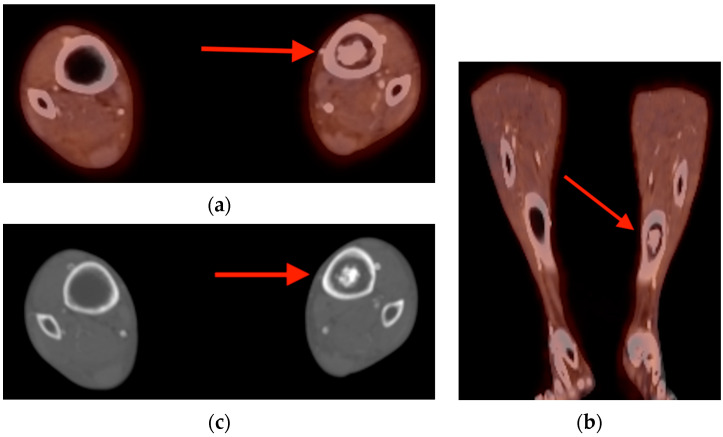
Axial (a) and coronal (b) fused FDG PET/CT images obtained in a 62-year-old man with melanoma showed a coarsely calcified intramedullary bone lesion with mild uptake within the left tibia (red arrows). (c) Plain CT images show the chondroid matrix calcifications and irregular but narrow zone of transition bordering this enchondroma in better detail (red arrow). CT = computed tomography. FDG PET/CT = fluorine-18 fluorodeoxyglucose positron emission tomography/computed tomography.
